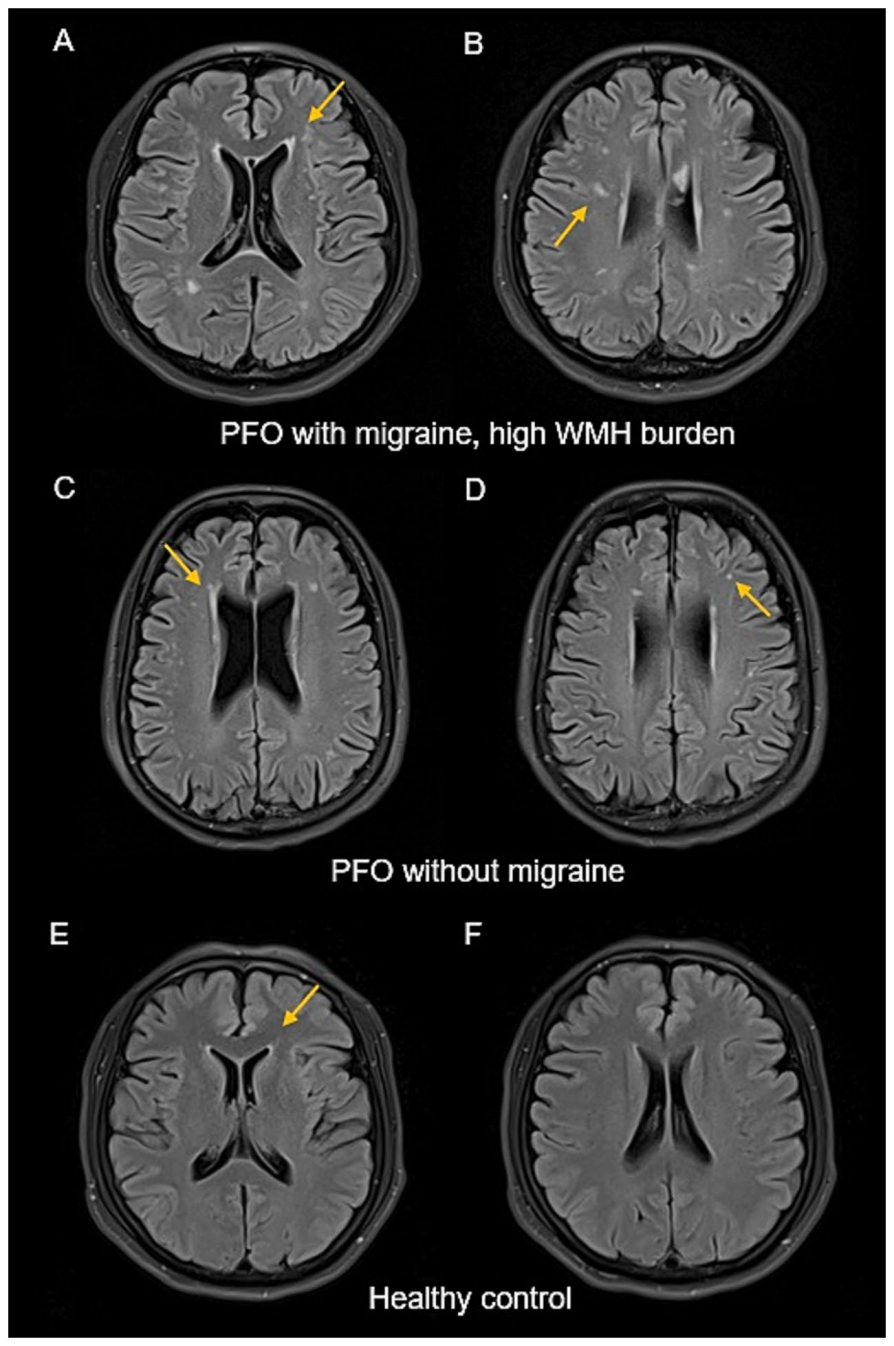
Representative images of WMH. FLAIR image of patient 1 with PFO: female, 50 years old, migraine, PFO diameter 3.3 mm, PVH Grade 2 (A) and DWMH Grade 3 (B). FLAIR image of patient 2 with PFO: male, 47 years old, without migraine, PFO diameter 2.0 mm, PVH Grade 2 (C) and DWMH Grade 1 (D). FLAIR image of HC: female, 49 years old, PVH Grade 1 (E) and DWMH Grade 0 (F). WMH, white matter hyperintensity; DWMH, deep white matter hyperintensity; HC, healthy control; PFO, patent foramen ovale; PVH, periventricular hyperintensity.
